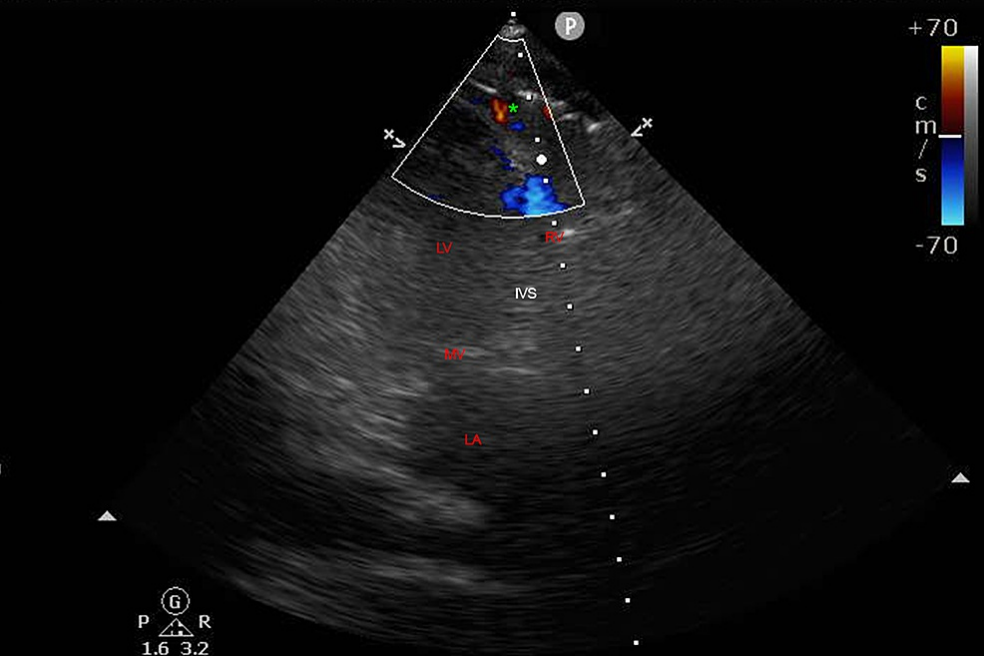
Color Doppler revealing extracardiac flow. Transthoracic echocardiographic view of extracardiac flow as indicated by green asterisk. RV: right ventricle; LA: left atrium; LV: left ventricle; MV: mitral valve; IVS: interventricular septum
